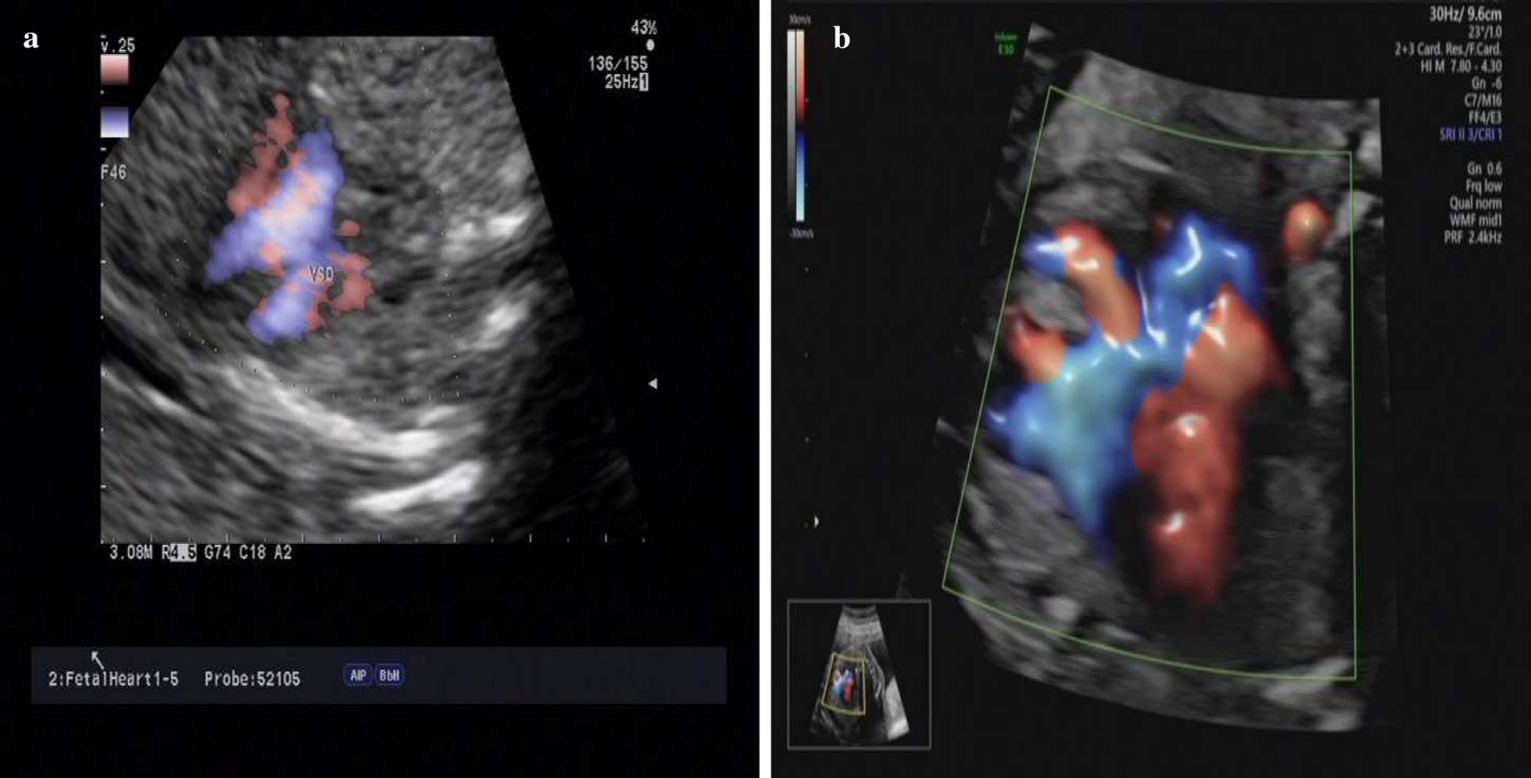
Ultrasound images of the fetuses. a An ultrasound image showed asymmetry of ventricular size with an enlarged right ventricle and a small left ventricle and ventricular septal defect in fetus 1 at 24 weeks of gestation. b An ultrasound image showed a complete atrioventricular septal defect in fetus 2 at 24 weeks of gestation
